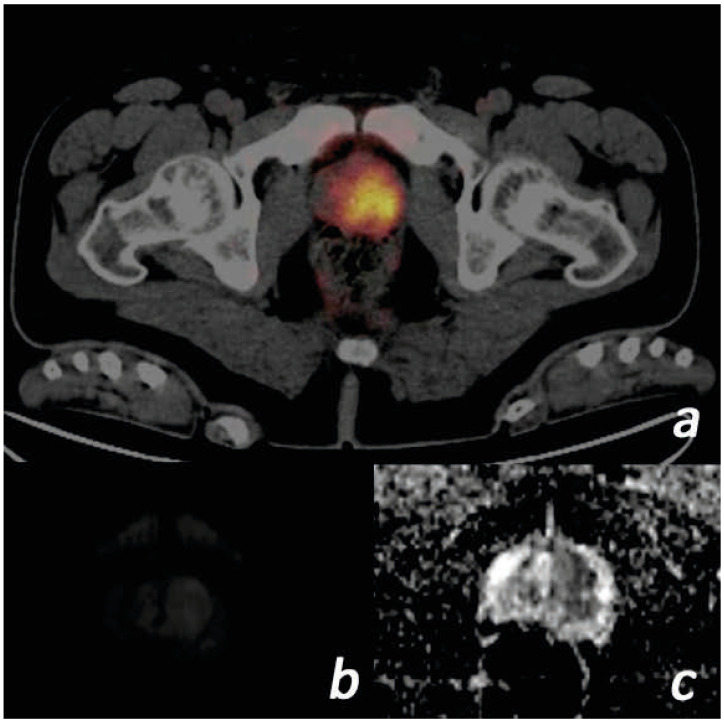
Images of a 57-year-old male patient. (PSA = 117.1 ng/mL, free PSA = 36.2 ng/mL, Gleason score = 4 + 3, ISUP grade = 3) (a) Tumor with SUVmax value of 16.38 on the left in the prostate gland transition zone on Ga-68 PSMA PET/CT focus is shown. (b) The lesion is slightly hyperintense on diffusion-weighted b = 800 images. (c) ADC images are markedly hypointense. The ADC value was measured as 0.40. ADC, apparent diffusion coefficient; ISUP, International Society of Urogenital Pathologists; PET/CT, positron emission tomography/computed tomography; PSA, prostate-specific antigen; PSMA, prostate-specific membrane antigen; SUVmax, standardized uptake value.
